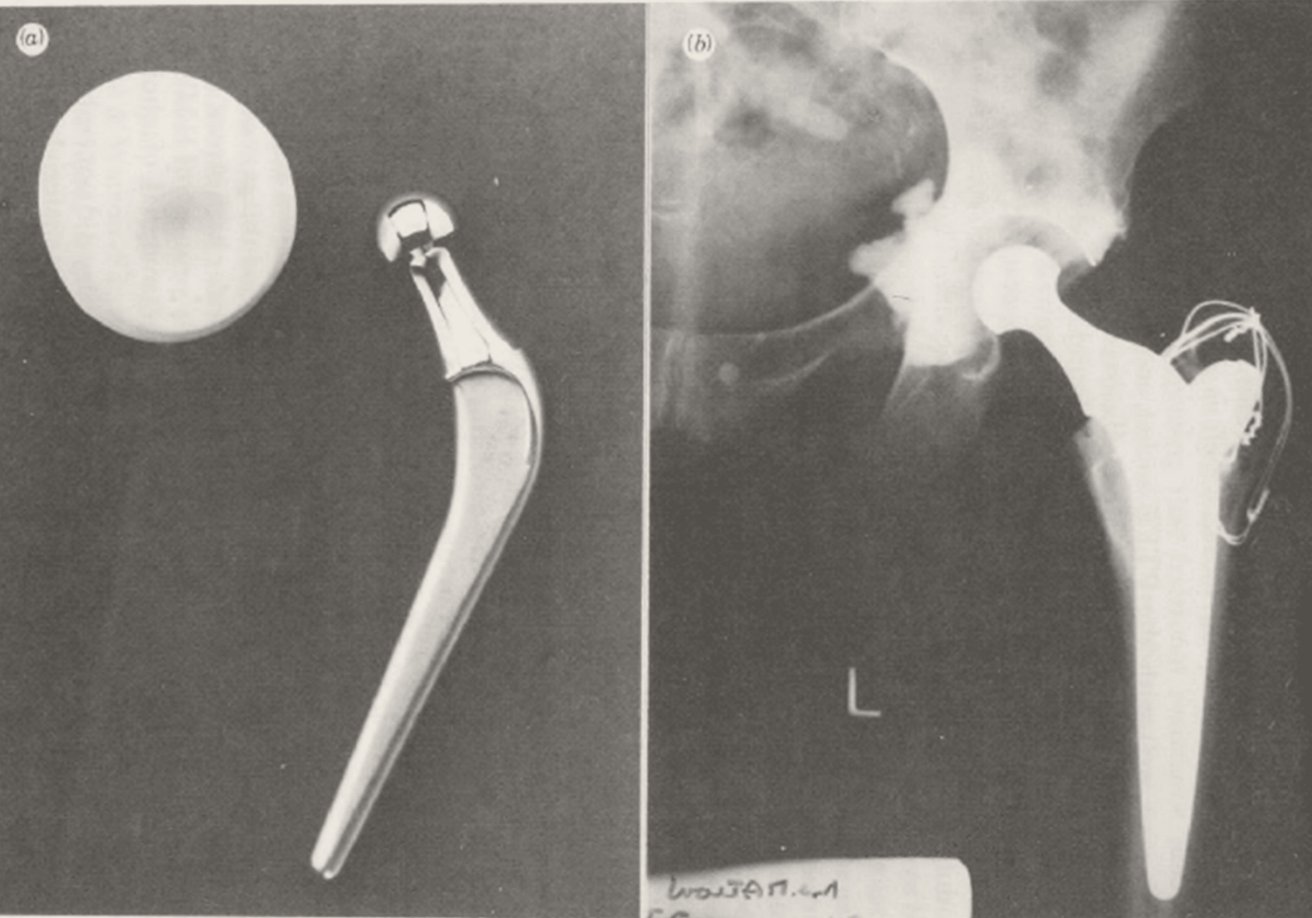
Dr. John H. Charnley's design of hip prosthesis (a) Charnley's design of low-friction arthroplasty prosthesis, (b) radiograph of the implanted prosthesis Image Credit: Charnley (open-access article) [6]
